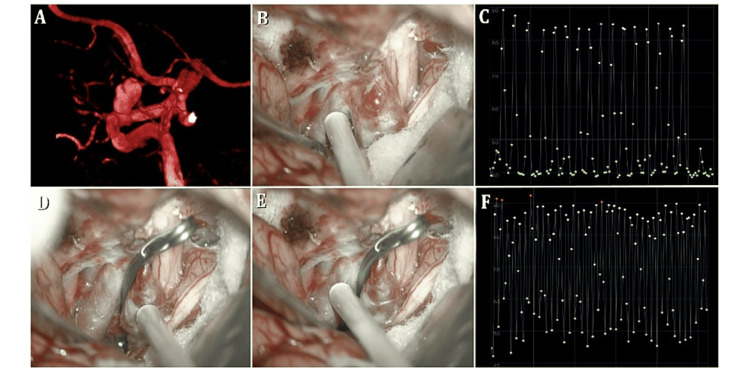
Patient one. (A) Ophthalmic carotid aneurysm on the left side. (B) The aneurysm is fully exposed after performing an anterior clinoidectomy. (C) Doppler decibelometry on the internal carotid in the post-aneurysm ophthalmic segment shows an average sound of 80 dB. (D) After clipping, we performed a Doppler on the aneurysm, which was negative. (E) We then placed the Doppler in the same location. (F) Doppler decibelometry shows the same sound intensity of 80 dB, confirming that the Doppler reading is exactly the same before and after clipping.
